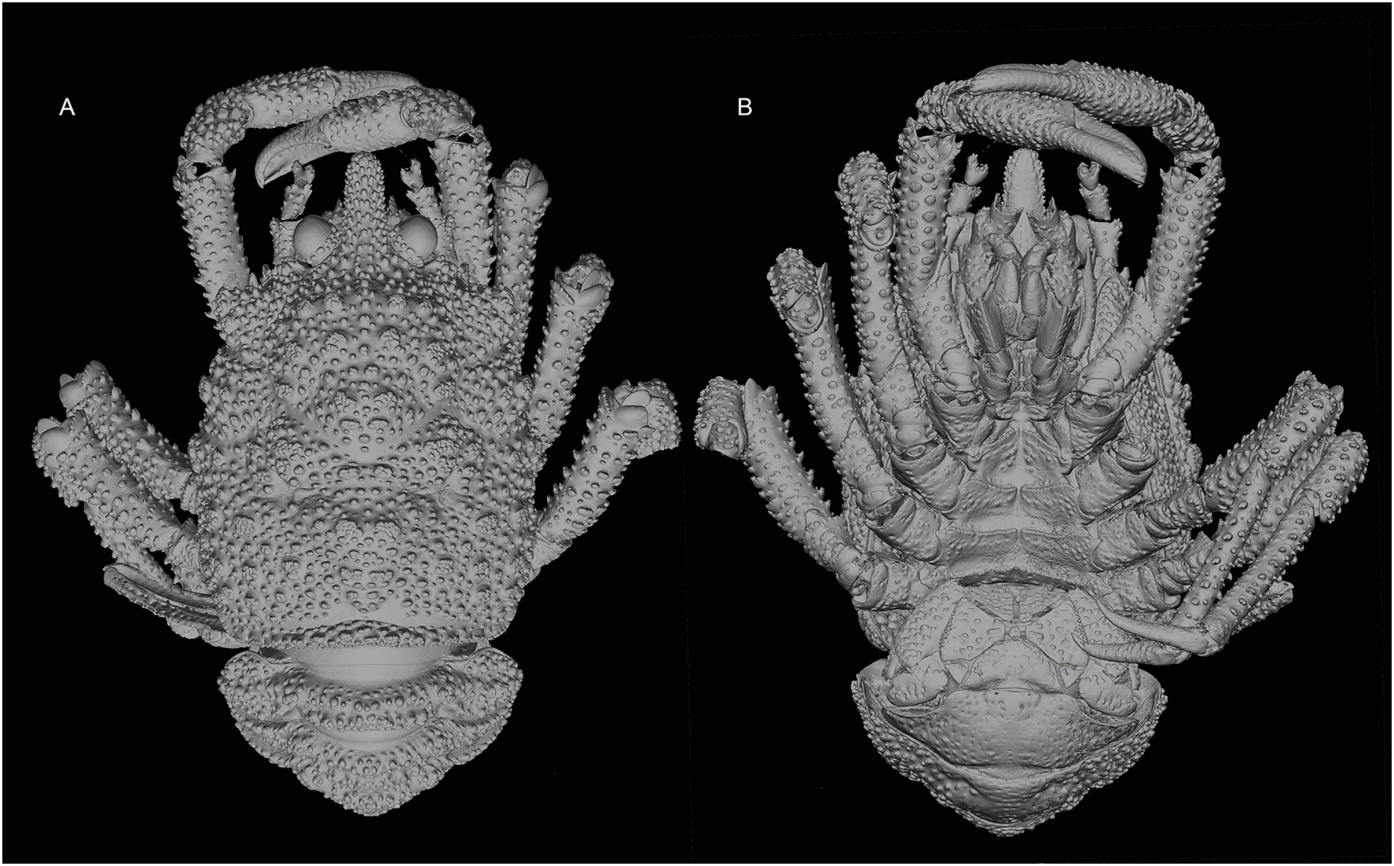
General habitus of the new species. 3D-renderings of micro-computed tomography x-ray images. *Munidopsis hawaii*
